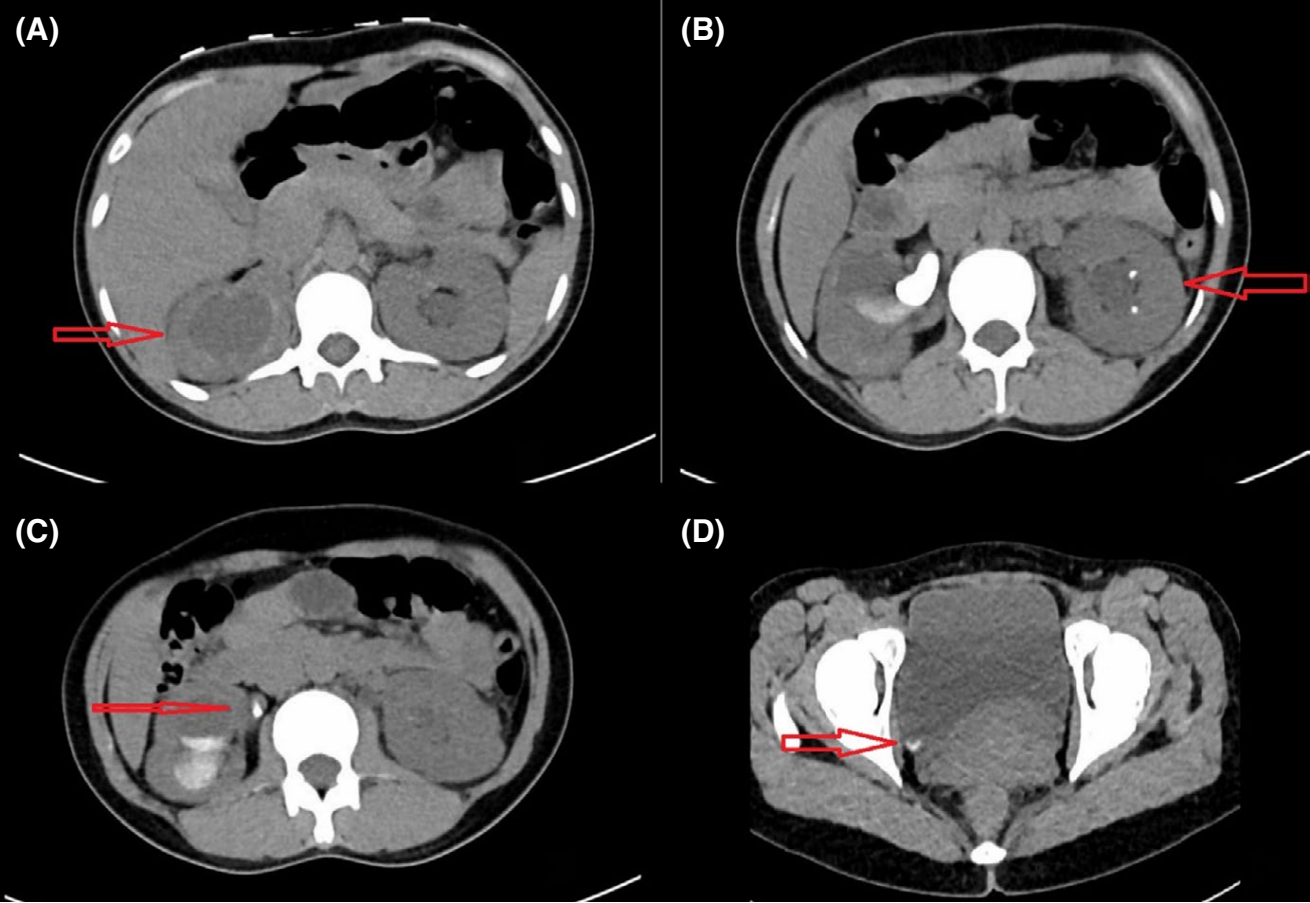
CT KUB findings of (A) Hydronephrosis, (B) Renal calculi with evidence of hydronephrosis, (C) Ureteric calculi, and (D) Urinary bladder stone
